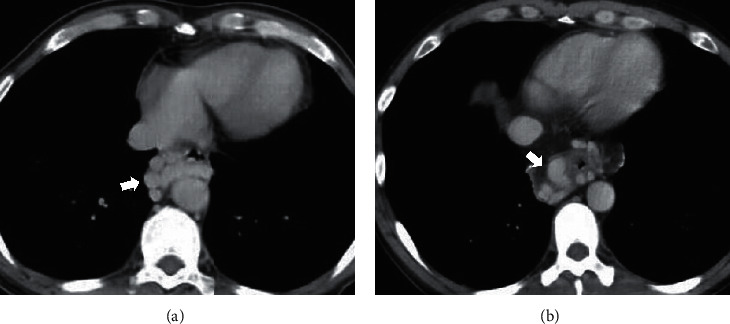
Esophageal collateral veins on contrast-enhanced CT scans.
